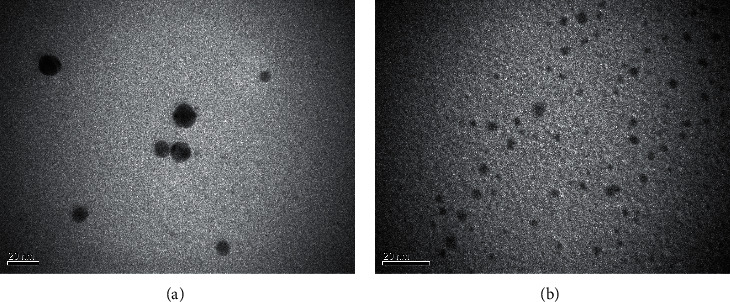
TEM images of AgNPs synthesized in solution NaPA (5 g L-1) by cyclic voltammetric (during 20 cycles) without ultrasonic field (a) and in the ultrasonic field (b), *t* = 20°C. Reproduced from [41] under the terms of the Creative Commons CC BY license.
